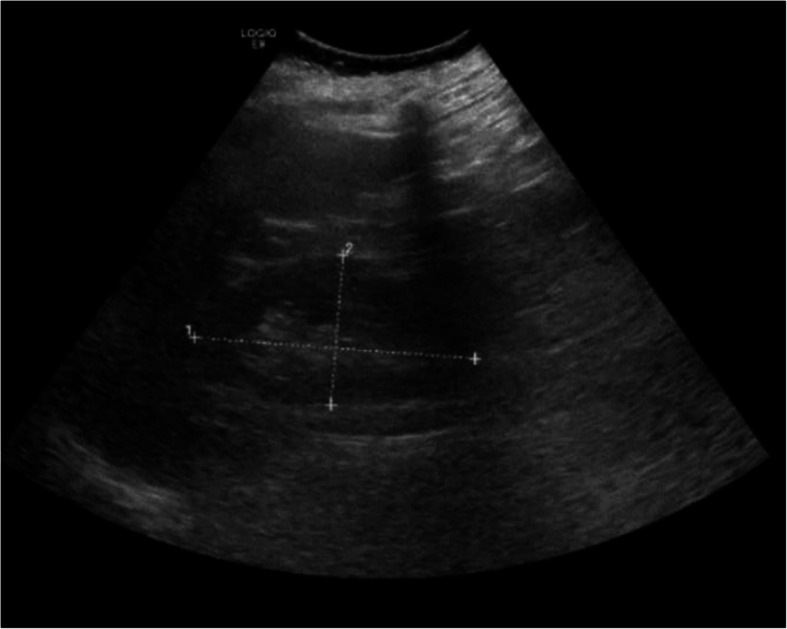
Ultrasound on arrival showing biliary sludge and cholelithiasis without any radiographic evidence of acute cholecystitis
